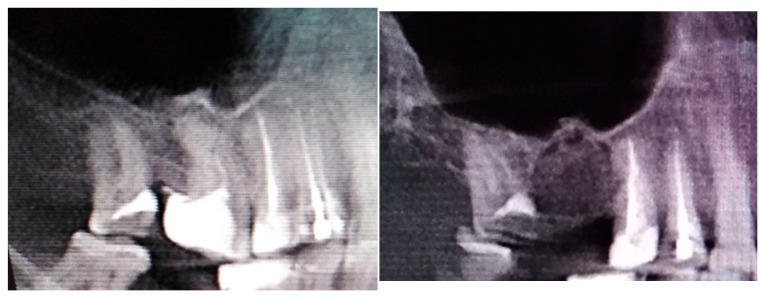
A case after the removal of the first upper right molar and curettage of some periapical inflamed tissue resulting in the loss of buccal cortical plate and opening of a huge oro-antral communication (CBCT scans in sagittal view).
